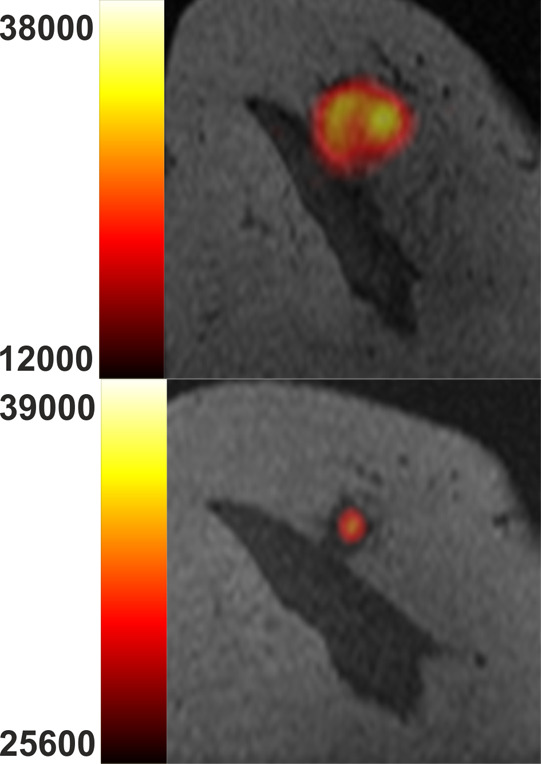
Multinuclear hydrogen 1 and ^13^C MR images of the right breast at baseline (top) and after one cycle of neoadjuvant chemotherapy (bottom). (a) Coronal summed hyperpolarized [1-^13^C]-pyruvate and (b) [1-^13^C]-lactate signal overlaid on unenhanced T1-weighted images. (c) Coronal dynamic contrast-enhanced MR image obtained 150 seconds after contrast agent injection and (d) overlaid transfer constant (*K*^trans^) map.
